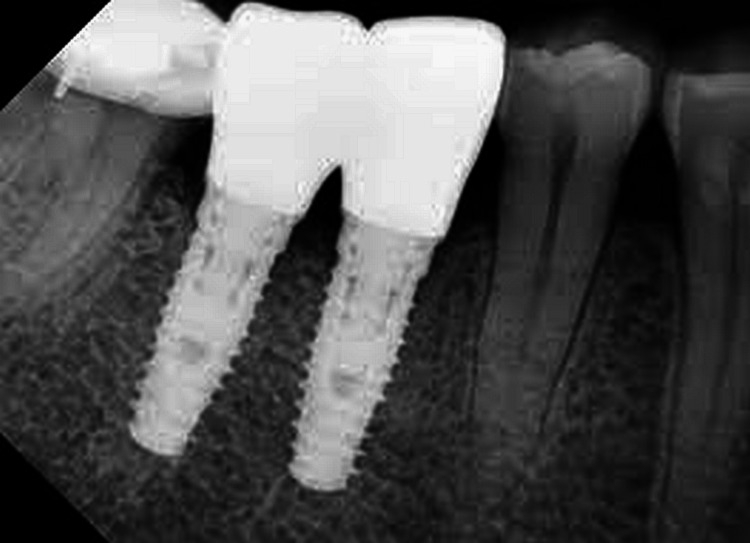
Implant placement radiograph
